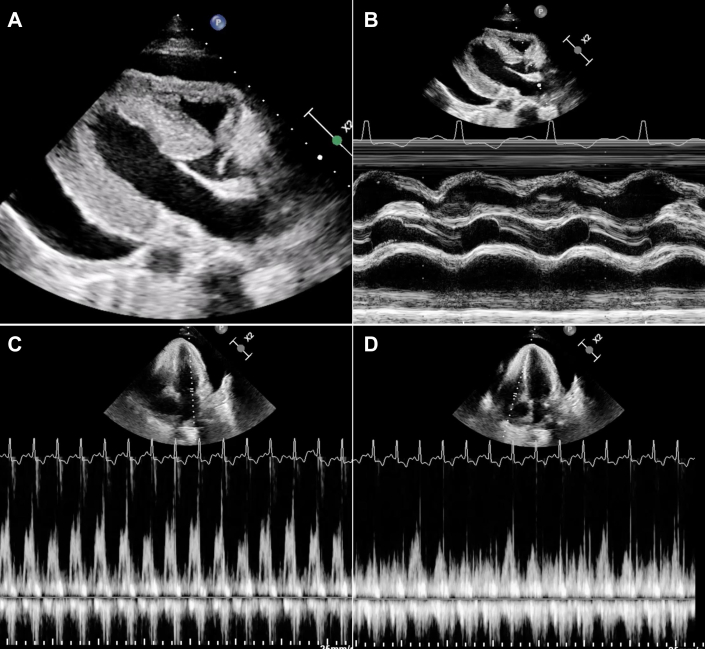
Transthoracic Echocardiogram Echocardiography demonstrated a large pericardial effusion on 2-dimensional imaging (A), with right ventricular diastolic collapse identified on M-mode imaging (B), and significant variation of mitral (C) and tricuspid valve inflow (D) on pulse-wave imaging.
